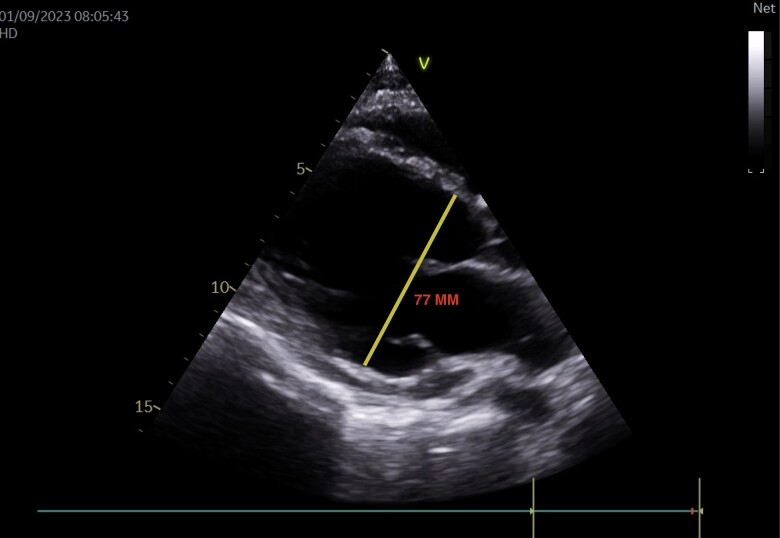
Parasternal long-axis echocardiographic section showing a dilated left ventricle with a telediastolic diameter of 77 mm.
